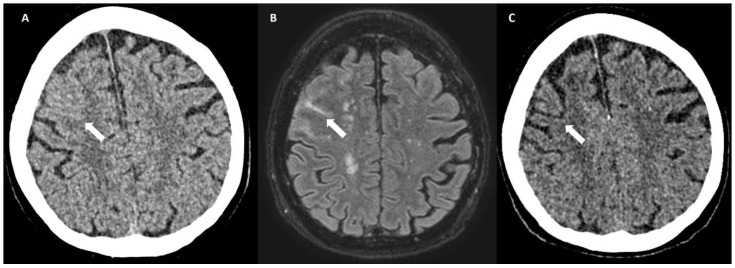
Right frontal subarachnoid hemorrhage on non-enhanced CT (A), 100 kV, 394 mAs, slice thickness 1 mm) and FLAIR MRI (B), 1.5 T, TE = 319 ms, TR = 4800 ms, TI = 1660 ms, slice thickness 3 mm) visible as the hyperdense/hyperintense effacement of the sulcus (A and B, white arrows). The control image ((C), non-enhanced CT, 100 kV, 394 mAs, slice thickness 1 mm) demonstrates the clearing of the sulcus (arrow).
